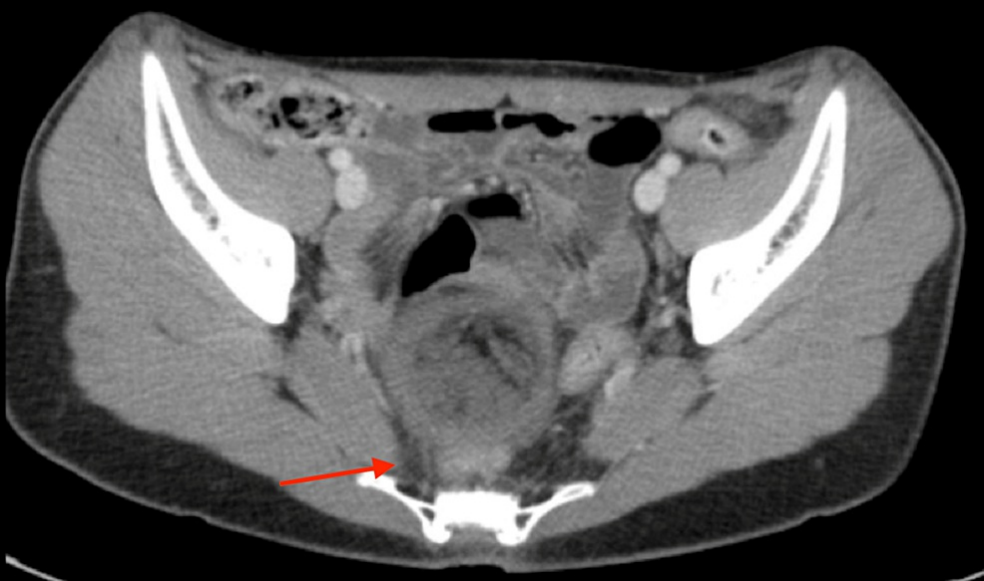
Axial view of the CT abdomen/pelvis showing distended loops of the bowel with compression of the bowel within the sigmoid colon (red arrow). CT: computed tomography
